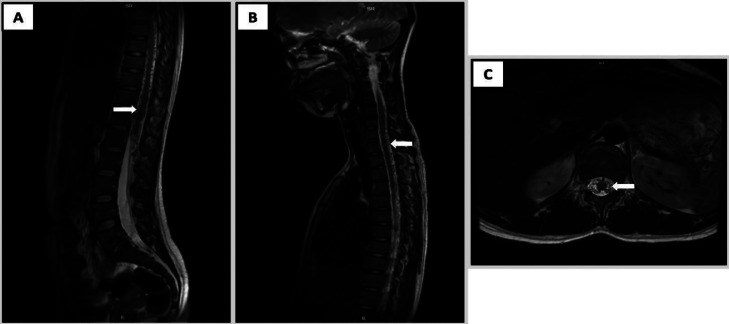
Pretreatment magnetic resonance (MR) images. (A) Sagittal T2-weighted MR image of the thoraco-lumbar spine showing vermiform vascular shadows extending from the thoracic to lumbar segments and spinal cord edema; (B) Sagittal T2-weighted MR image of the cervico-thoracal segment showing perimedullary flow void (C) Axial T2-weighted MR image showing dilatation of perimedullary vein in thoracal level.
